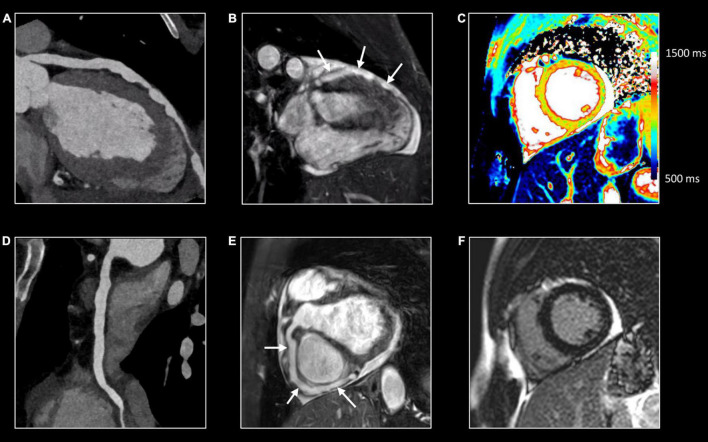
Comprehensive imaging in coronaritis. Twenty-seven-year-old man with systemic vasculitis, likely adult-onset Kawasaki disease), admitted with non-STEMI. Computed tomography (CT) (A,D) and CMR (B,E) of the coronary arteries show irregular aneurysmatic dilation (up to 8 mm) of the coronary arteries with on the left anterior descending (LAD) coronary artery several skip areas (focal pseudostenoses). Although the CMR was requested because of clinical suspicion of non-STEMI, CMR revealed no myocardial edema (normal T1/T2 mapping) (C) nor abnormalities at LGE imaging (F) suggestive of myocardial necrosis and/or fibrosis. Further investigation showed a normal caliber of the thoraco-abdominal aorta. However, aneurysms were found on several middle-sized abdominal arteries, including the celiac trunk, common hepatic artery, superior and inferior mesenteric artery and both renal arteries.
